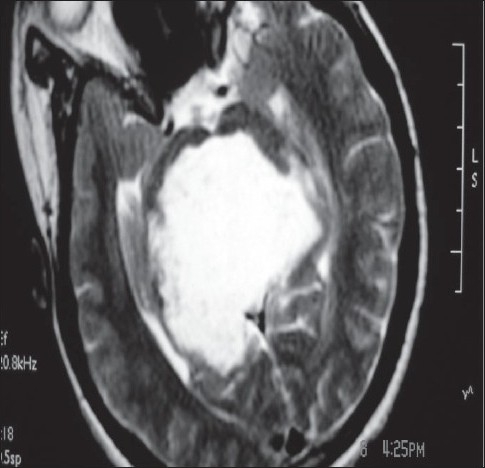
Postoperative T1-weighted axial magnetic resonance imaging showing near-total excision of the tumor; the wall is left behind
